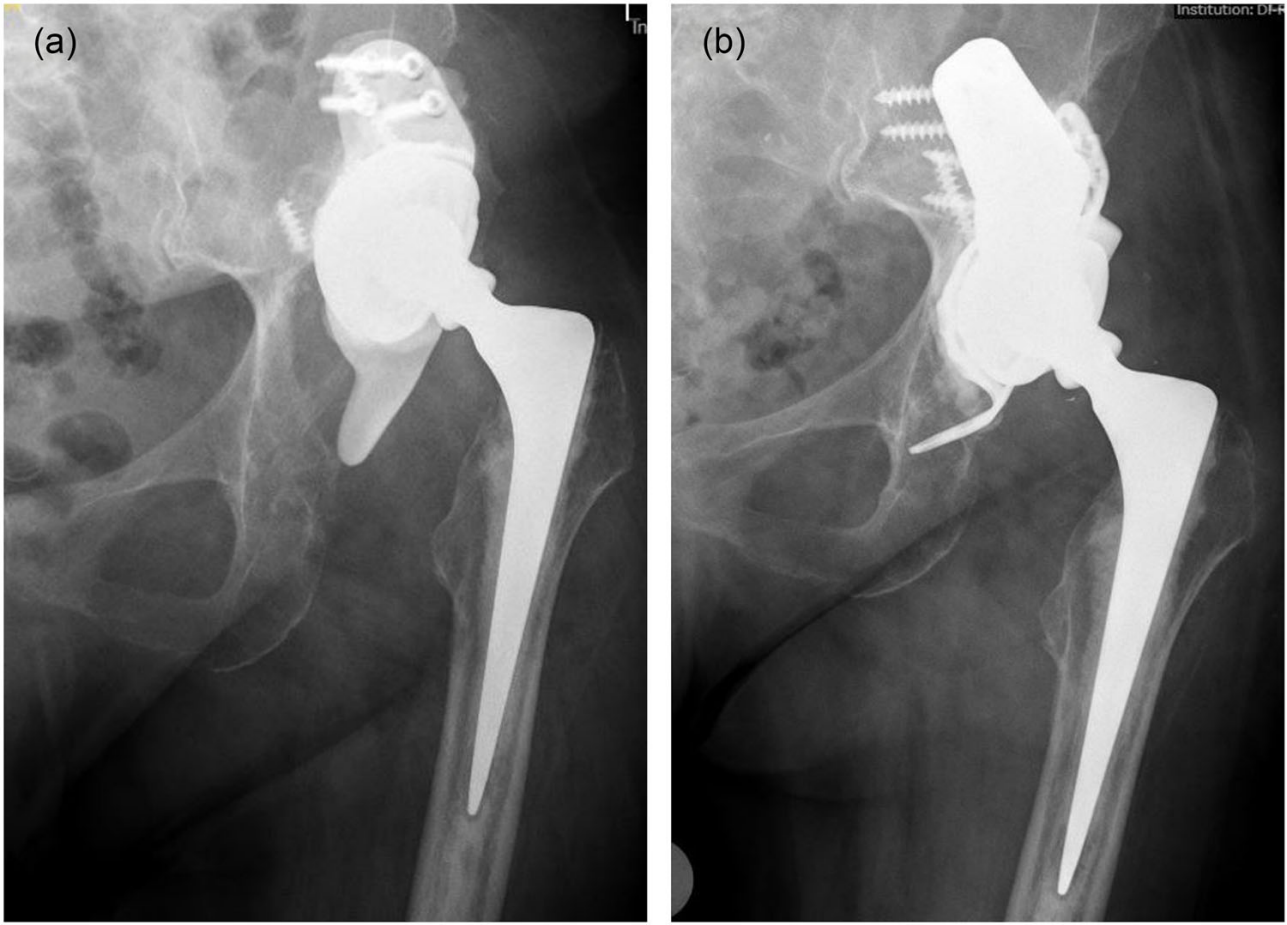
(a) Preoperative anteroposterior radiograph of the hip of an 80‐year‐old patient with severe acetabular bone loss due to aseptic loosening of the cup construct. (b) Anteroposterior radiograph of the same patient postoperative 4.0 years after surgery using a modular tantalum TM buttress augment in combination with a Contourring and a cemented dual‐mobility cup. There are no signs of loosening of one of the components. TM, trabecular metal.
